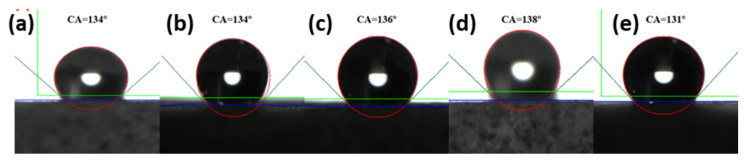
The contact angle of the treated core sheet after ultrasonic cleaning. (a–e) the contact angles on the treated core sheet after being cleaned with pure water for 5 min, 10 min, 20 min, 30 min, and 45 min.
